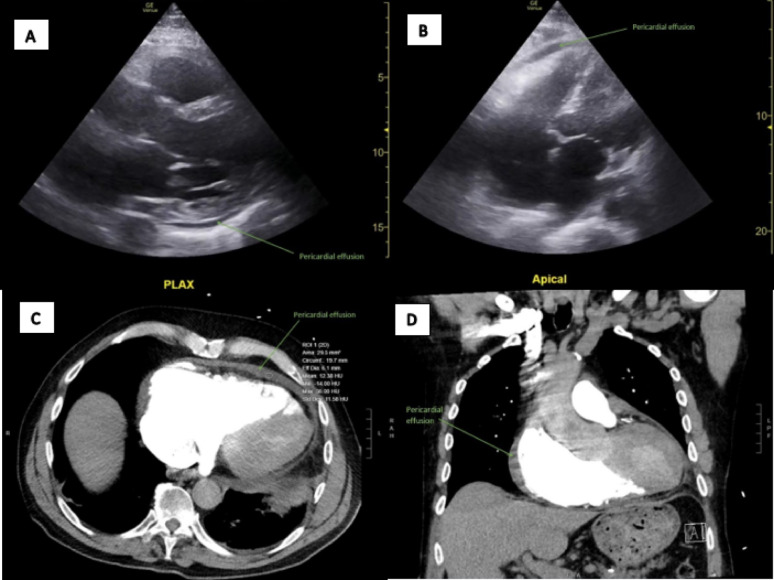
Panels A and B. Point-of-care cardiac ultrasound still image, parasternal long axis and apical four chamber views, showing a small to moderate pericardial effusion and dilated cardiomyopathy (B-mode). Panels C and D. Axial and coronal chest computed tomography scan with intravenous contrast showing a small to moderate pericardial effusion with mild pericardial fat stranding
